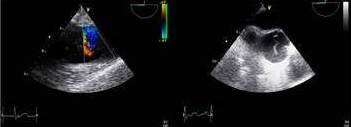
Transesophageal echocardiogram revealing intimal flap in ascending aorta.
